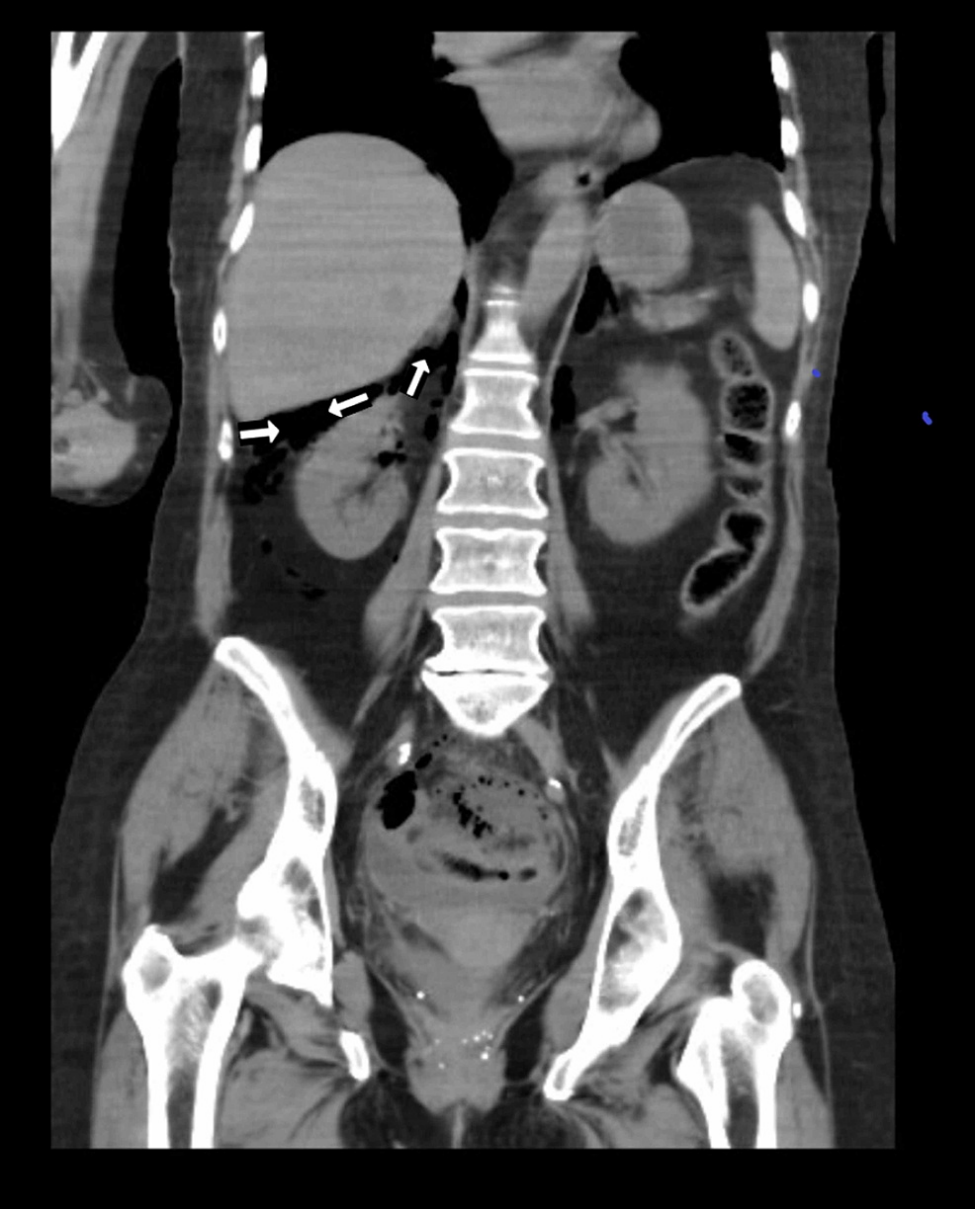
CT Scan Abdomen and Pelvis without contrast- Coronal View- Showing air under the liver from intestinal perforation.
